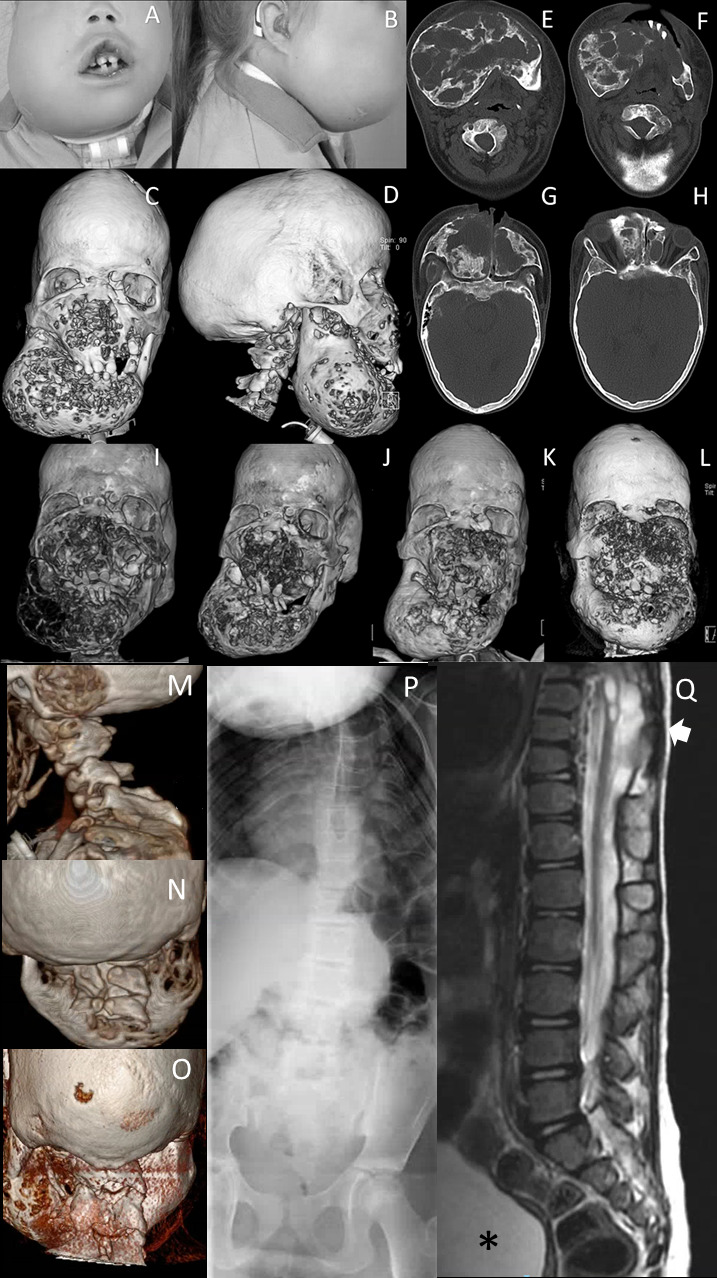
Clinical and radiological facial features of subject 1. (A, B) Facial photographs at age 11. Dysmorphic features include frontal bossing, dolichocephaly, hypertelorism, half-closed eyes, a broad and flat nasal bridge, and an asymmetric bilateral swelling at mandibular and mid-facial levels. (C, D) 3-D reconstructed CT images of the face at age 11 years show a bilateral asymmetric cystic expansion of the mandible, maxilla, ethmoid and frontal bones with medial displacement of the teeth at mandibular level. In addition, frontal bossing and dolichocephaly can be noted. (E–H) Transverse CT images of at mandibular and mid-facial levels at age 11 years show extensive osteolytic and osteoblastic lesions. Note that the lesions are not only at mandibular level but also on mid-facial level and the anterior side of the foramen magnum. (I) 3-D reconstructed CT image of the face at age 9 years shows a bilateral asymmetric cystic expansion of the mandible, maxilla, ethmoid and frontal bones with medial displacement of the teeth and bilateral orbital involvement. (J) One year after the start of pamidronate (age 11 years), small cystic lesions can be seen at mandibular and mid-facial level; note the difference in osseous tissue compared with the CT scan before the start of the therapy. (K) Five years after the start of therapy (age 14 years), more remodelling of osseous tissue has occurred, although small cystic lesions are present. (L) One year after stopping pamidronate and 2 years after shaving approximately 2.5 cm of the right maxilla (age 17 years), multiple cystic lesions at mandibular and mid-facial levels with intensive displacement of the orbita. CT 3-D reconstruction of the skull, with (M) a sagittal view and (N) a posterior view of the cervical spine showing, besides lytic lesions, abnormalities of the cervical vertebrae at the age of 6 years and (O) at 18 years. (P) Spine X-rays at the age of 7 years showing scoliosis and abnormal thoracic vertebrae. (Q) Sagittal T2-weighted MRI of the thoracolumbal spine showing the thoracic syrinx and meningomyelocele (arrow) and neurogenic bladder (asterisk).
